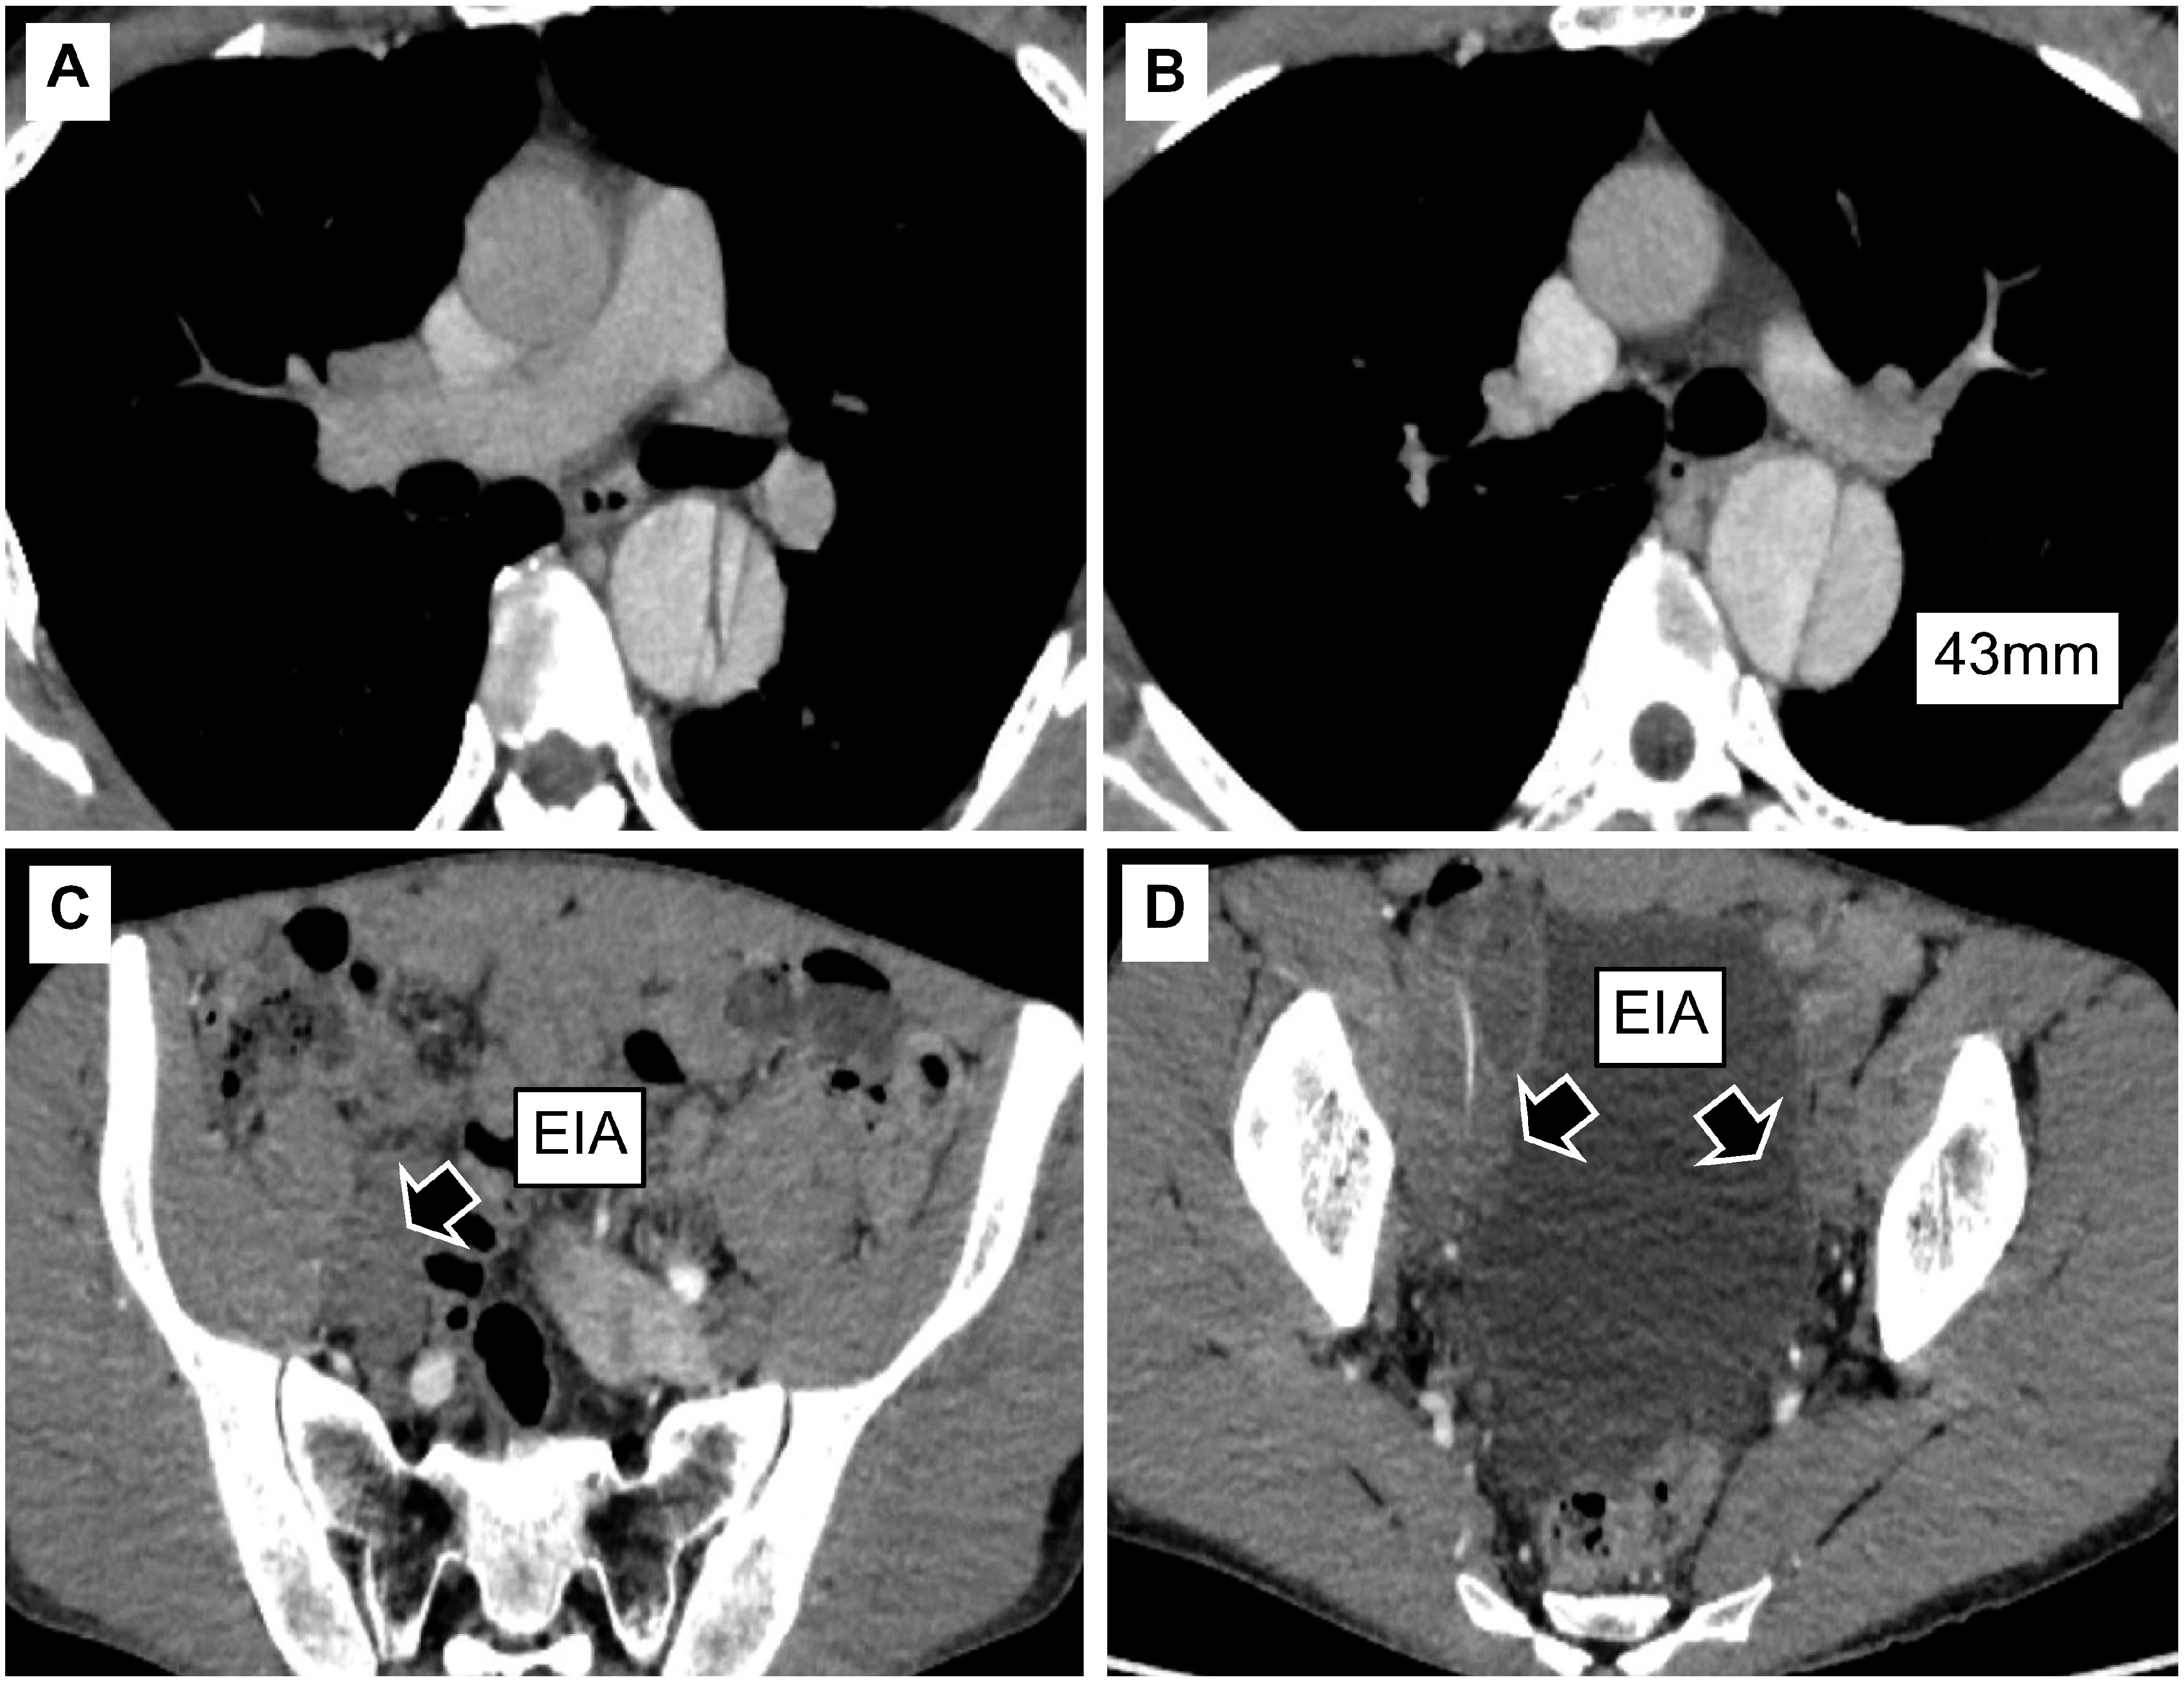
Fig. 3 (A) Computed tomography showing the second Stanford type B acute aortic dissection after 16 months since the onset of the first aortic dissection. (B) The maximum axial diameter of the descending aorta was 43 mm. (C) The blood flow in the external iliac artery (EIA) was disrupted. Arrow: The left EIA. (D) Disrupted blood flow of the left and right EIAs.
